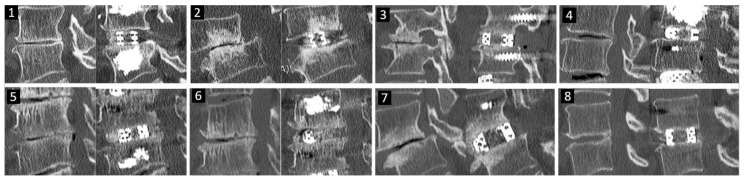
Eight different patients’ pre and post-operative mid sagittal cut CT scan showing the increase in the intervertebral disc height.
